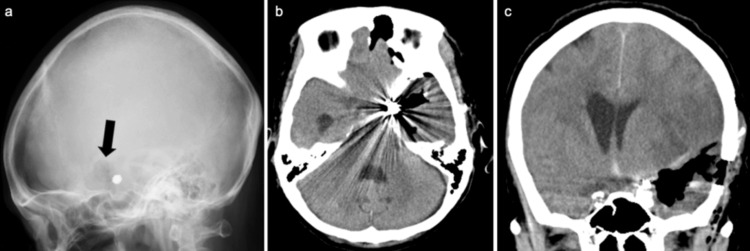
X-ray and CT following endoscopic hematoma evacuation (a) Lateral X-ray showing the burr hole location (arrow). (b, c) Axial and coronal CT scans confirming successful hematoma removal.
